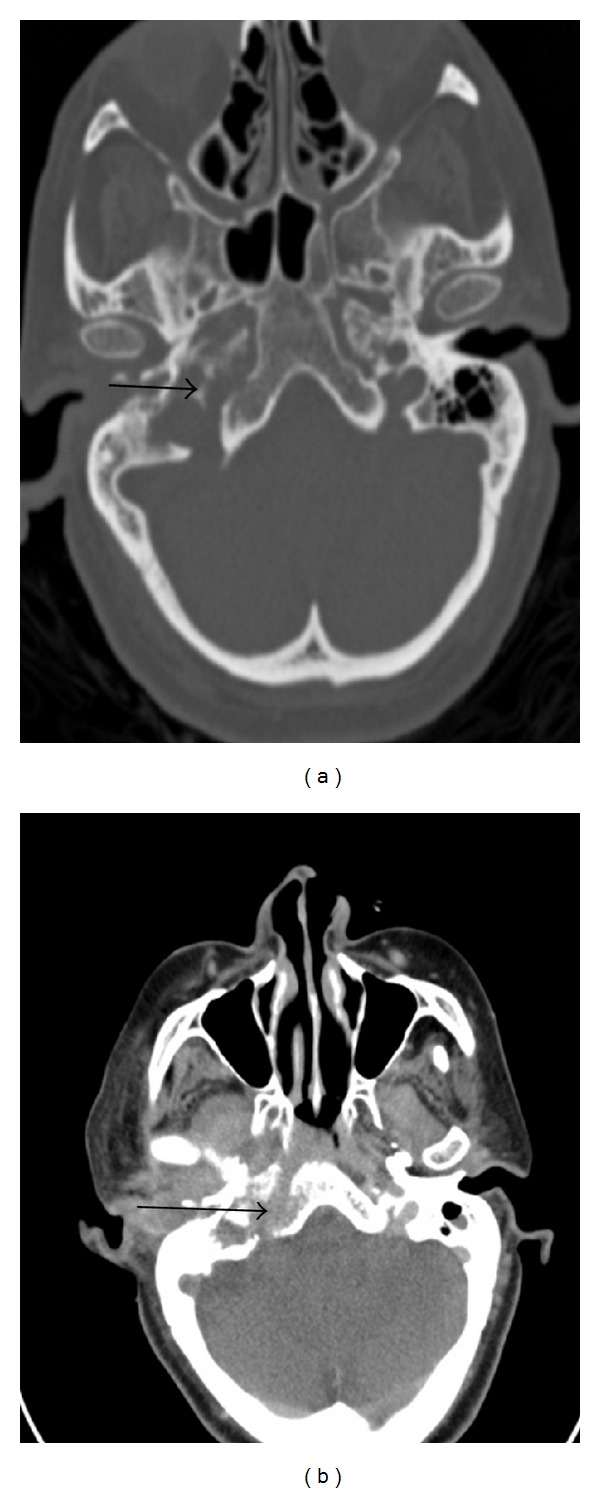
Right inner ear and temporal bone computed tomography scan on first admission (a) and on readmission (b) showing opacification of the right external auditory canal, right tympanic, and epitympanic spaces, as well as the right mastoid antrum. There is also bony destruction of the anterior wall of the right external auditory canal, scutum and wall of the right carotid canal, foramen lacerum, and semicircular canals. The arrows show the extent of tumor spread into the middle ear.
